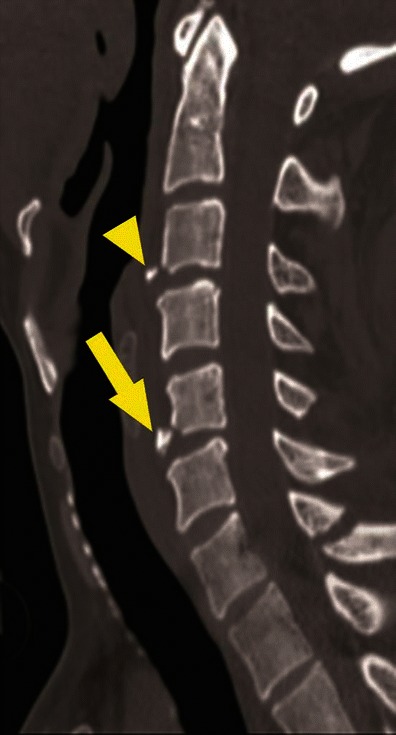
Hyperextension teardrop. Sagittal CT image shows small triangular avulsion fractures at the anteroinferior end plate of C3 (*arrowhead*) and C5 (*arrow*) without significant prevertebral soft tissue lesion. The triangular fragment at C5 is taller than wider, suggestive of hyperextension teardrop than hyperextension dislocation
